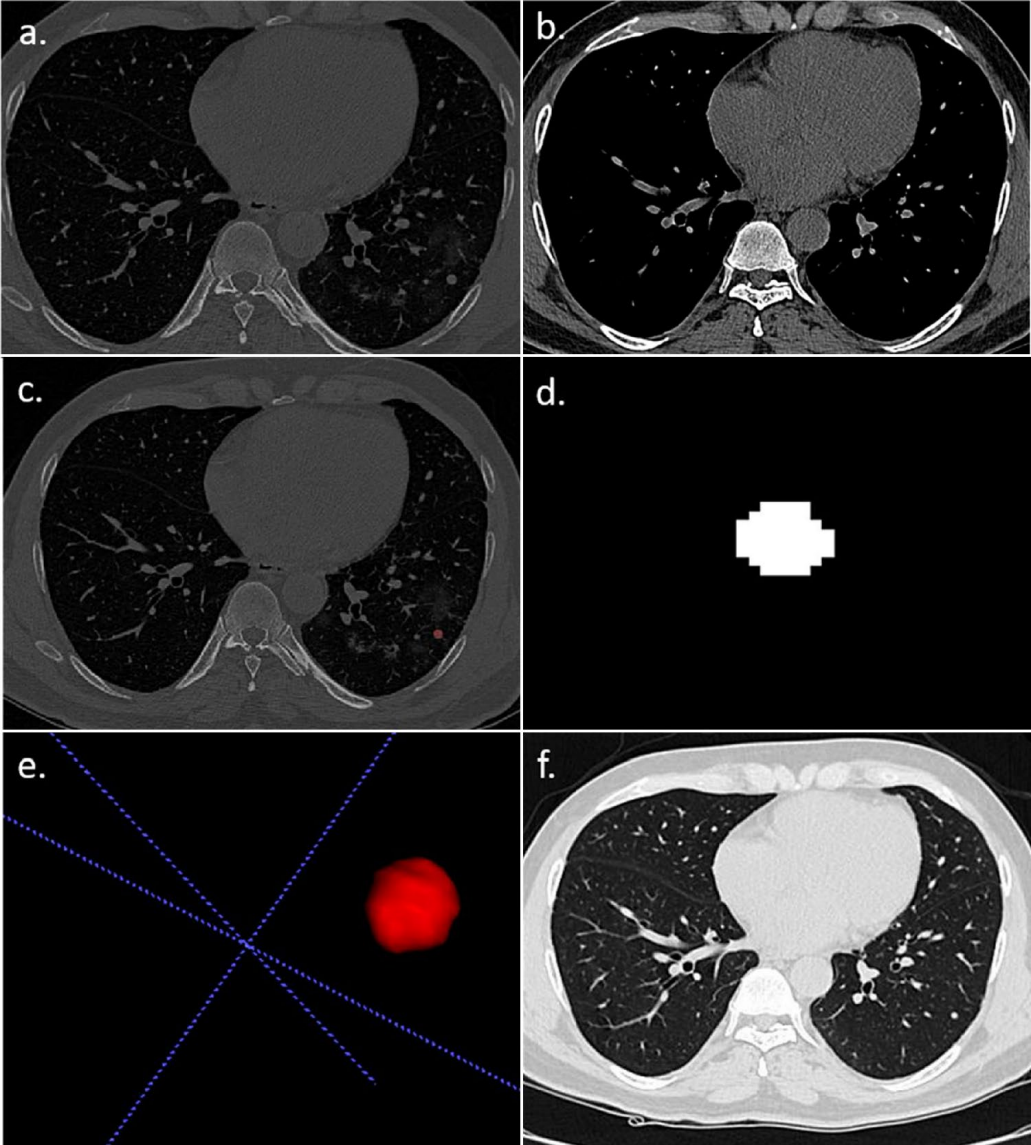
A 57 year-old male patient with RT–PCR has proven COVID-19 pneumonia. (a,b) The axial section of the CT thorax in the lung window and soft tissue window shows a subpleural soft tissue nodule in the posterior segment of the left lower lobe. (c) Creation of ROI for segmentation. (d) 2D-segmented nodule. (e) 3D-volumetric rendering of the nodule. (f) Follow-up chest CT after 6 months revealed partial resolution of the nodule.
